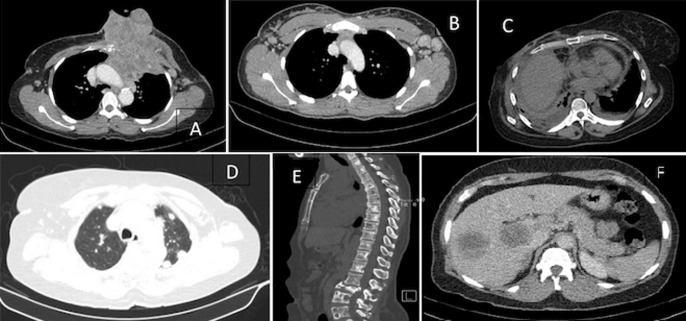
axial computed tomography scan images of various patterns of breast cancer metastases: A) direct extension of the disease to the anterior mediastinum; B) lymph node metastasis-bilateral enlarged and infiltrated axillary lymph nodes; C) bilateral malignant pleural effusion and pericardial effusion; D) multiple metastatic lung nodules; E) osteolytic, osteoblastic and mixed bone metastatic patterns; F) liver metastasis - hypodense nodules which enhance less than normal liver parenchyma
